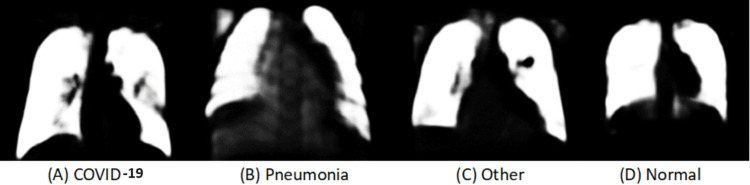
(A-D) Segmented chest x-ray images
